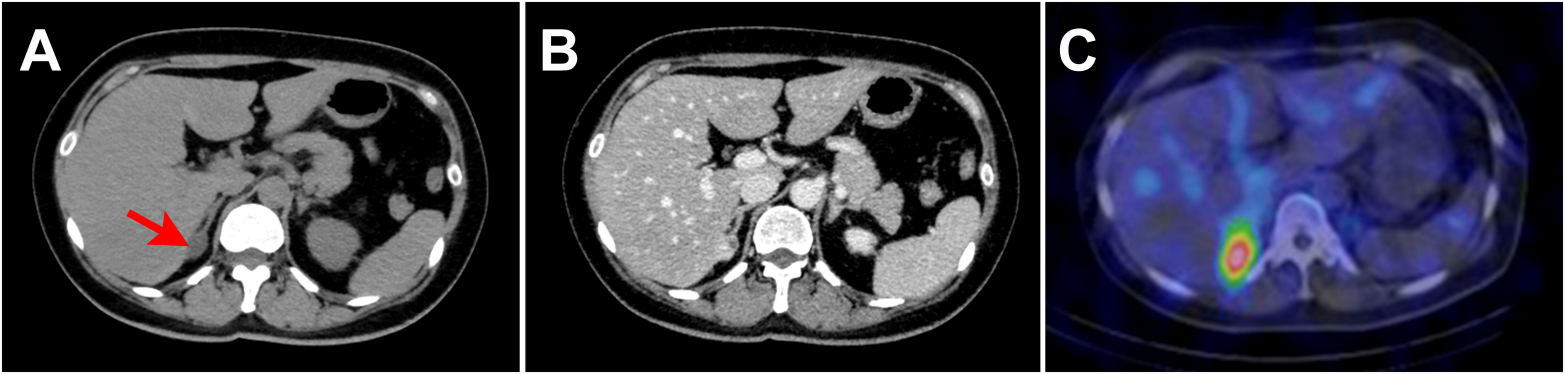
Imaging findings. (A, B). Computed tomography (CT) revealed a 2 cm right adrenal mass (red arrow in (A)). The mean Hounsfield unit of the adrenal tumor on unenhanced CT was 14.0. (A). Unenhanced CT. (B). Contrast enhanced CT. (C). NP-59 scintigraphy with dexamethasone suppression showed increased tracer uptake in the right adrenal lesion.
